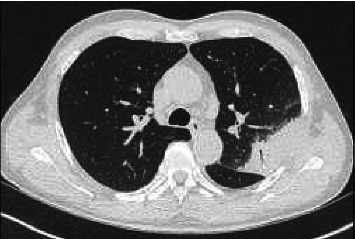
Chest CT scan showing subpleural lobar condensation in the left upper lobe with air bronchogram.
